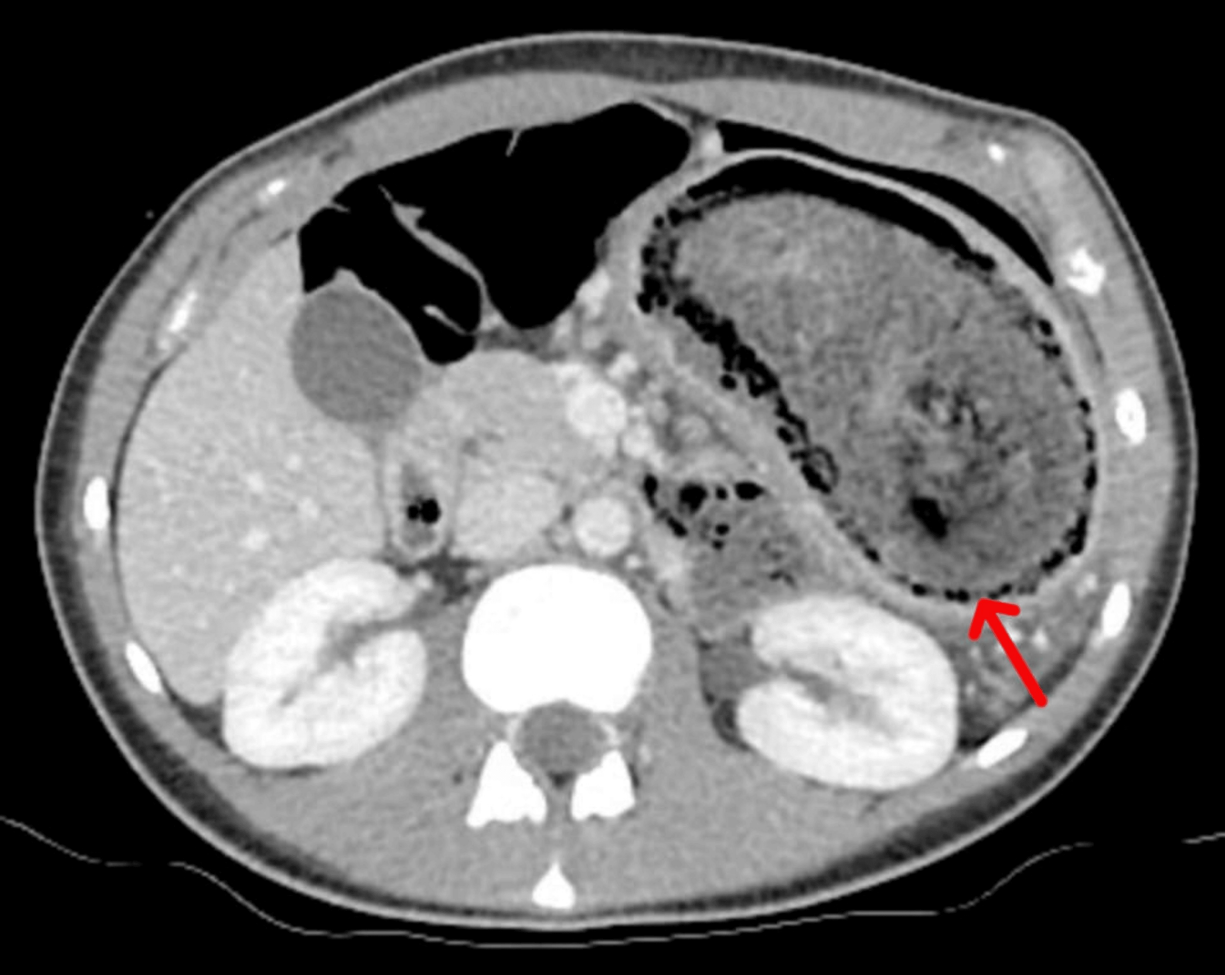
CECT whole abdomen (Axial section) showing heterogeneous intraluminal mass in the stomach, with a mottled gas pattern (Red arrow) CECT: Contrast-enhanced computed tomography
